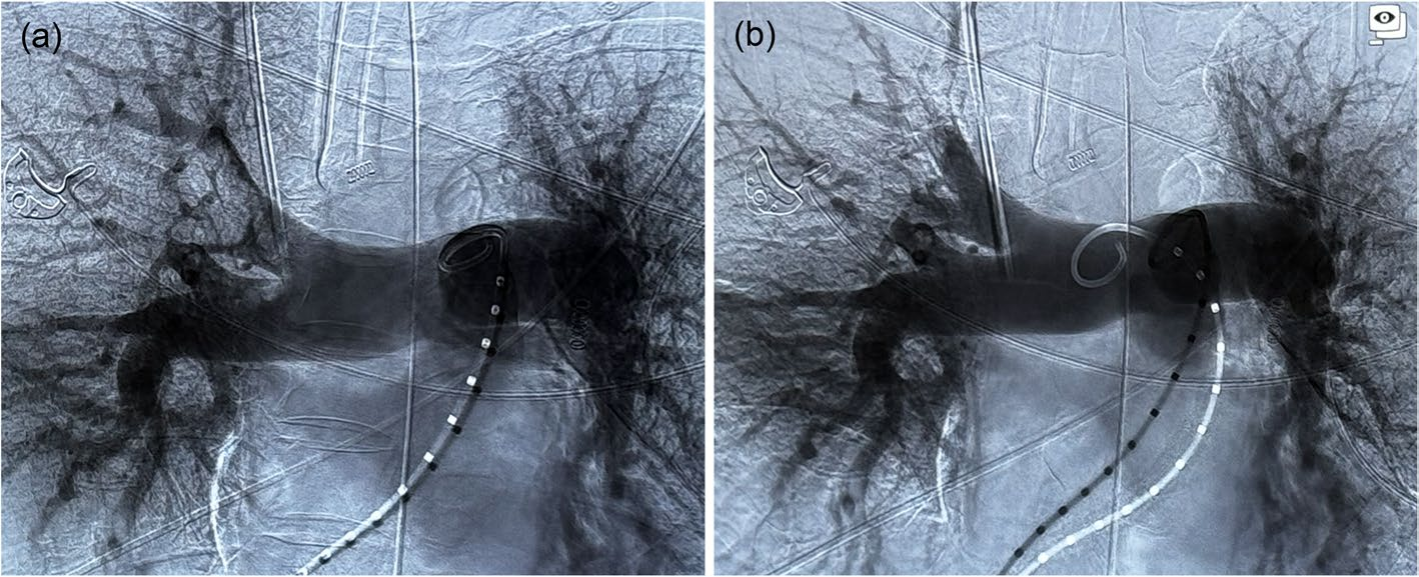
Pulmonary angiograms taken before and after large-bore mechanical thrombectomy (a) Pre-thrombectomy angiogram confirming right main and right upper lobe filling defects corresponding to CT pulmonary angiogram findings. (b) Post-thrombectomy angiogram demonstrating resolution of central emboli with residual small volume subsegmental right upper lobe emboli
